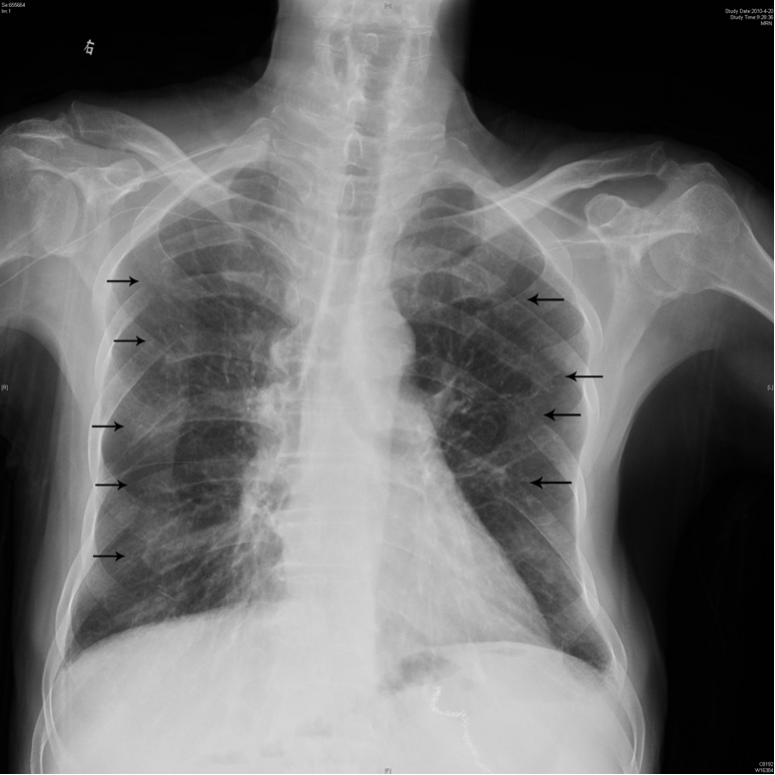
X-ray image. A chest radiograph showed multiple fractures of the left fourth to seventh ribs (arrow) and right fifth to ninth ribs (arrow).
